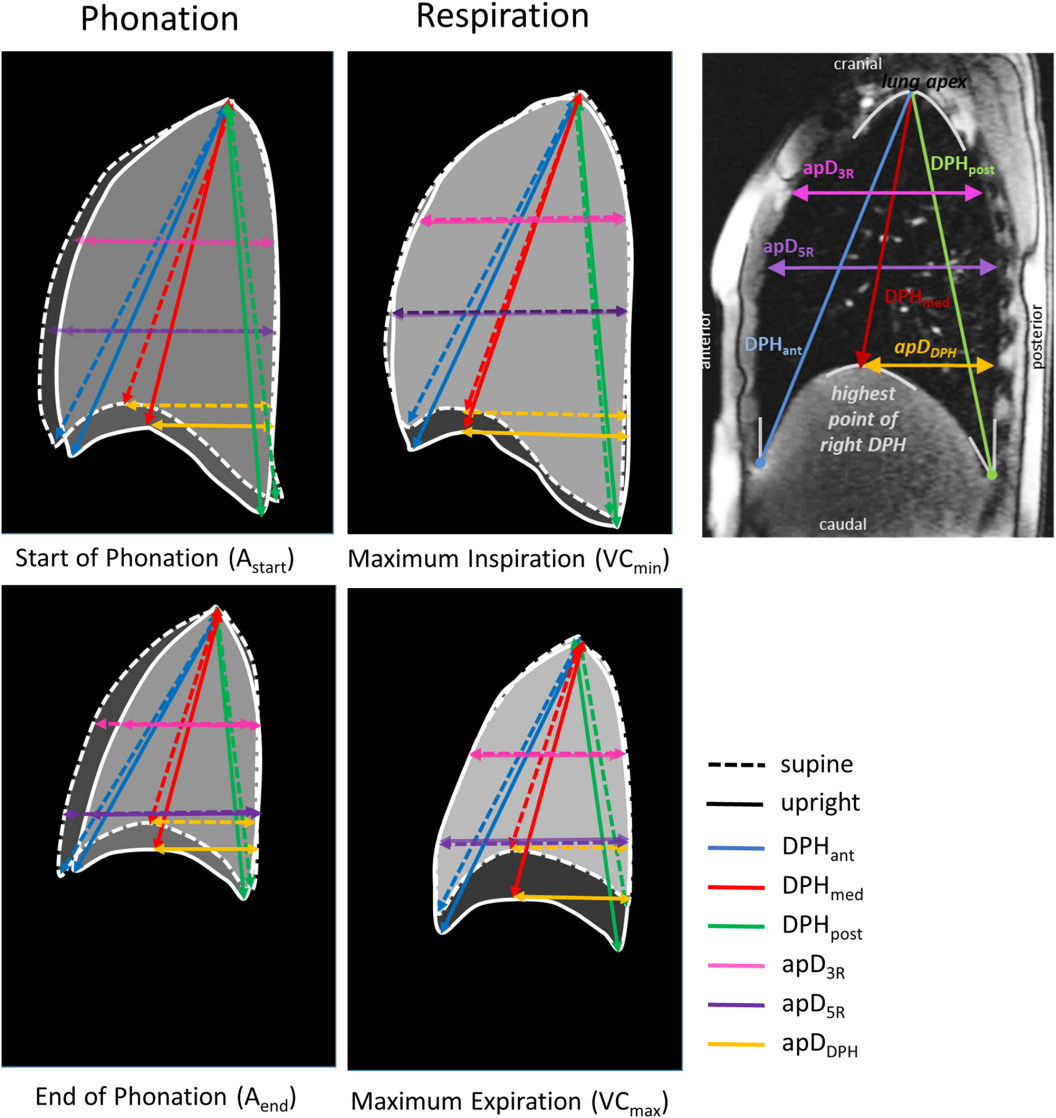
Exemplary visualisation of differences in lung dimensions of subject 3 derived from MRI data at the start and end of phonation of A4 as well as maximum inspiration and expiration in the upright and supine positions. The dimensions of the right lung in sagittal cross-section are shown as a grey area on a black background and differentiated for the upright and supine body positions based on the outline (dashed = supine, solid = upright). Measured distance parameters are displayed in different colours according to Fig. 2.
